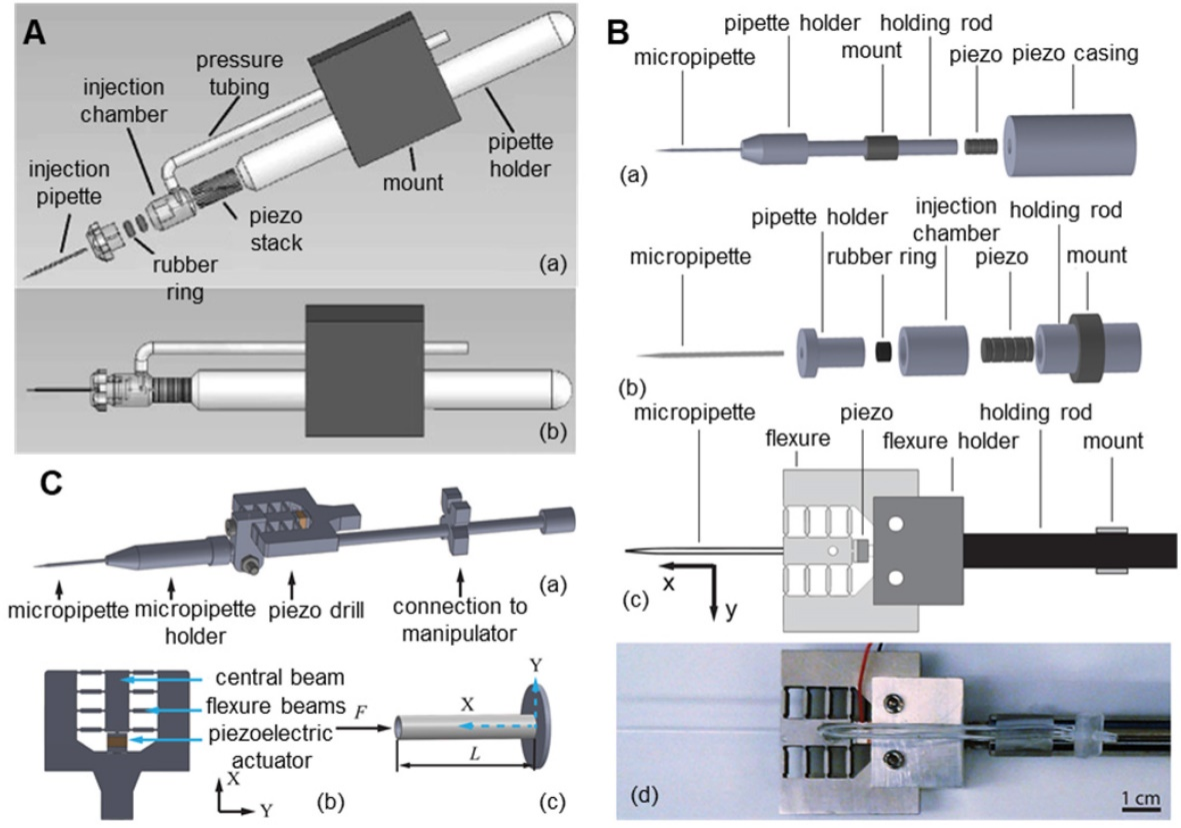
Various improved piezoelectric ultrasonic microinjectors. (A) Microinjector with piezoelectric ceramic front. (B) Piezoelectric ultrasonic microinjector with a flexible mechanism. (C) Microinjector with piezoelectric actuator eccentric configuration. Adapted with permission from 104, copyright 2011 Spring Nature, and 106, copyright 2018 IEEE, and 107, copyright 2020 IEEE.
